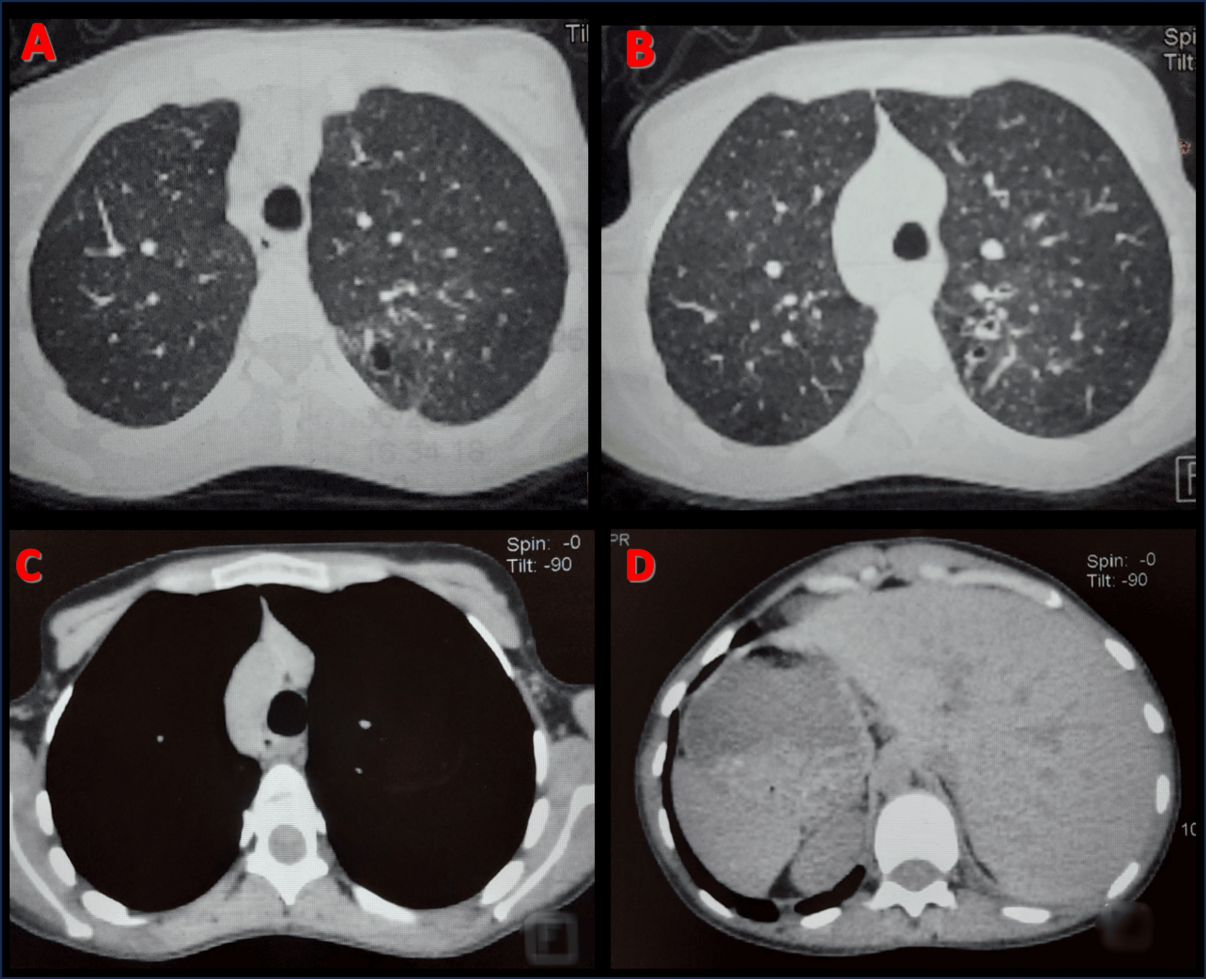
Thoracoabdominal CT scan Thoracoabdominal CT revealed cystic bronchiectasis (A and B), in the setting of complete situs inversus (trilobed left lung, bilobed right lung, transposed mediastinal vessels, right aortic arch, left-sided liver, and right-sided spleen) (C and D).
